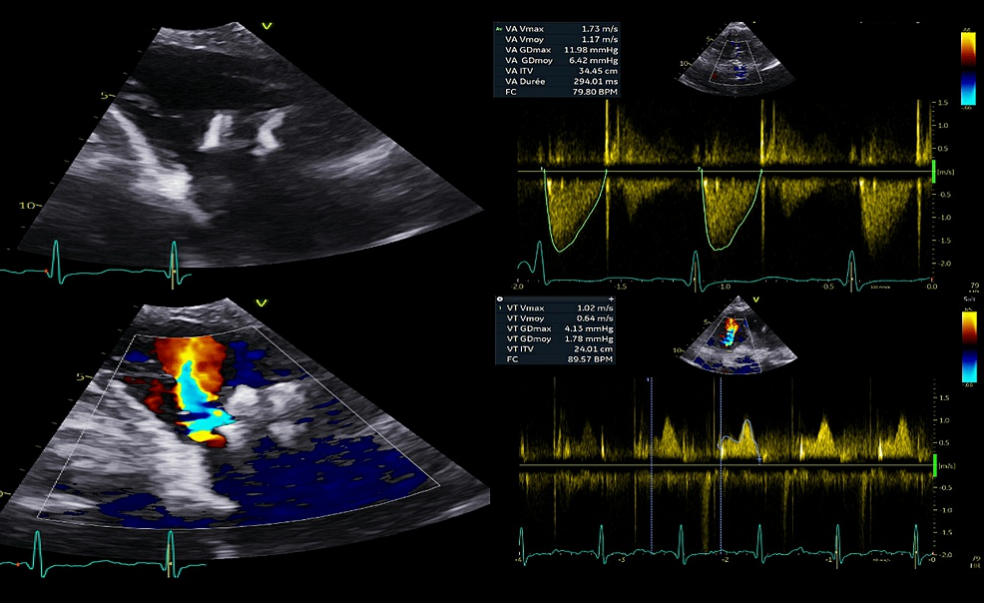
Postprocedural echocardiogram showing a well-functioning TAVI and TEER TAVI: transcatheter aortic valve implantation; TEER: transcatheter edge-to-edge tricuspid valve repair
